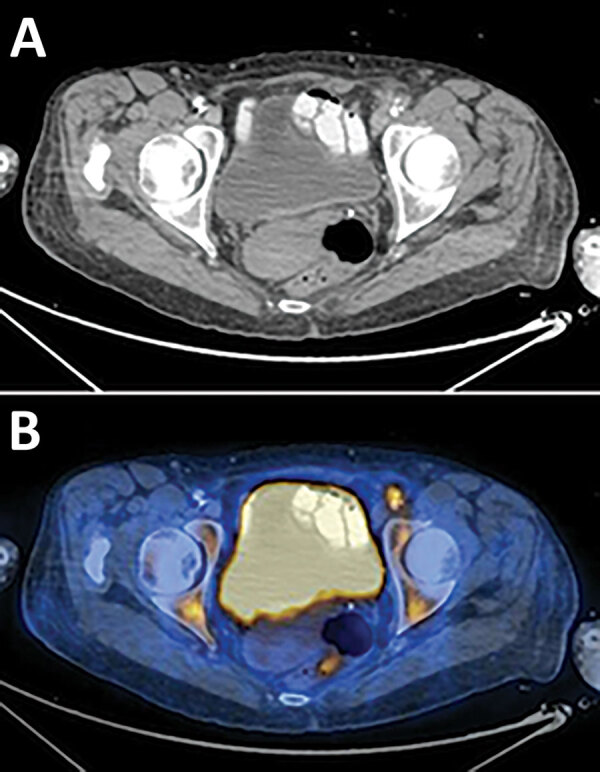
Imaging of an immunocompromised patient who had endovascular infection with *Kingella kingae* complicated by septic arthritis, Israel. A) Computed tomography scan shows a luminal stenosis in the transition zone of the left external iliac artery and common femoral artery along with surrounding turbid fat. B) Fluorodeoxyglucose positron emission tomography–computed tomography scan showing high fluorodeoxyglucose uptake in the plaque causing the stenosis.
